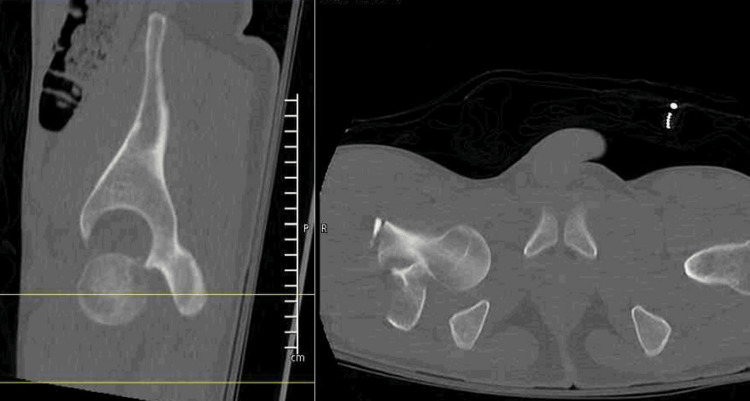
Sagittal and corresponding axial CT image of the right hip showing inferior hip dislocation The femoral head sits directly inferior to the acetabular lip without anterior or posterior displacement.
